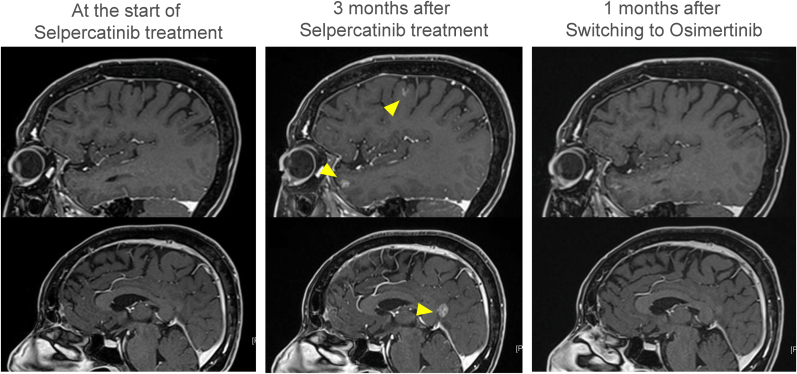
Sagittal images of brain enhanced magnetic resonance imaging. No enhanced lesion was observed at the start of selpercatinib treatment, but 3 months after starting selpercatinib, lesions with contrast effects appeared (yellow arrowheads) and enhancement images of the cerebral sulci were observed. One month after switching to osimertinib treatment, these contrast effects disappeared.
